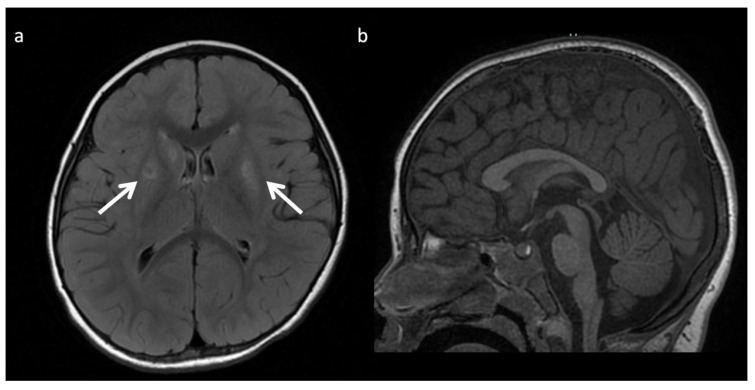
Pyruvate dehydrogenase complex (PDHc) deficiency in a 30-month-old female with desaturations. (a) Axial T2 FLAIR image shows a Leigh pattern of brain injury with bilateral putamen and right caudate head (white arrows) hyperintense lesions with central signal suppression representing necrosis. (b) Sagittal T1WI shows mild pontine hypoplasia and thinning of the callosal splenium, likely representing regional hypogenesis rather than volume loss, given low normal callosal length and normal cerebral white matter depth.
